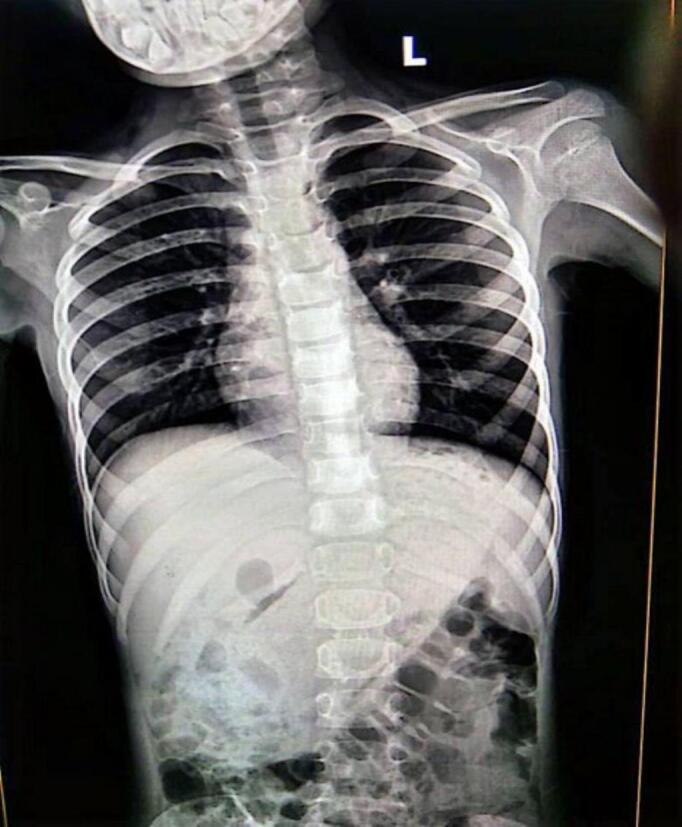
This is an AP view X-ray of the chest and abdomen showing clear lung fields, a normal cardiac silhouette, and no bony deformity There is a well-defined intragastric soft tissue density mass is visible, projecting over the left upper quadrant and mid-abdomen with mottled lucencies, consistent with a trichobezoar. No evidence of perforation or bowel obstruction is observed.
